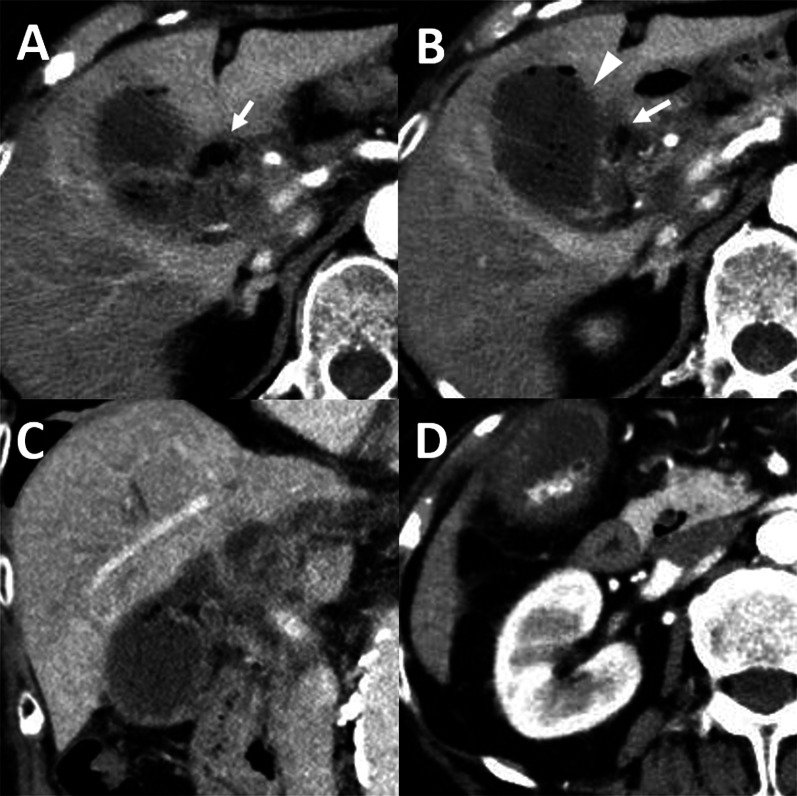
Enhanced CT images of the gallbladder and peri-gallbladder tissues. A, B CT showed gas formation in the gallbladder lumen and wall that spread to the hepatoduodenal ligament (arrows) and the decreased enhancement of the gallbladder wall (B arrowhead). C Coronal-view CT showed fluid collection within the hepatoduodenal ligament. D CT showed accumulated small gallstones in the fundus of the gallbladder
